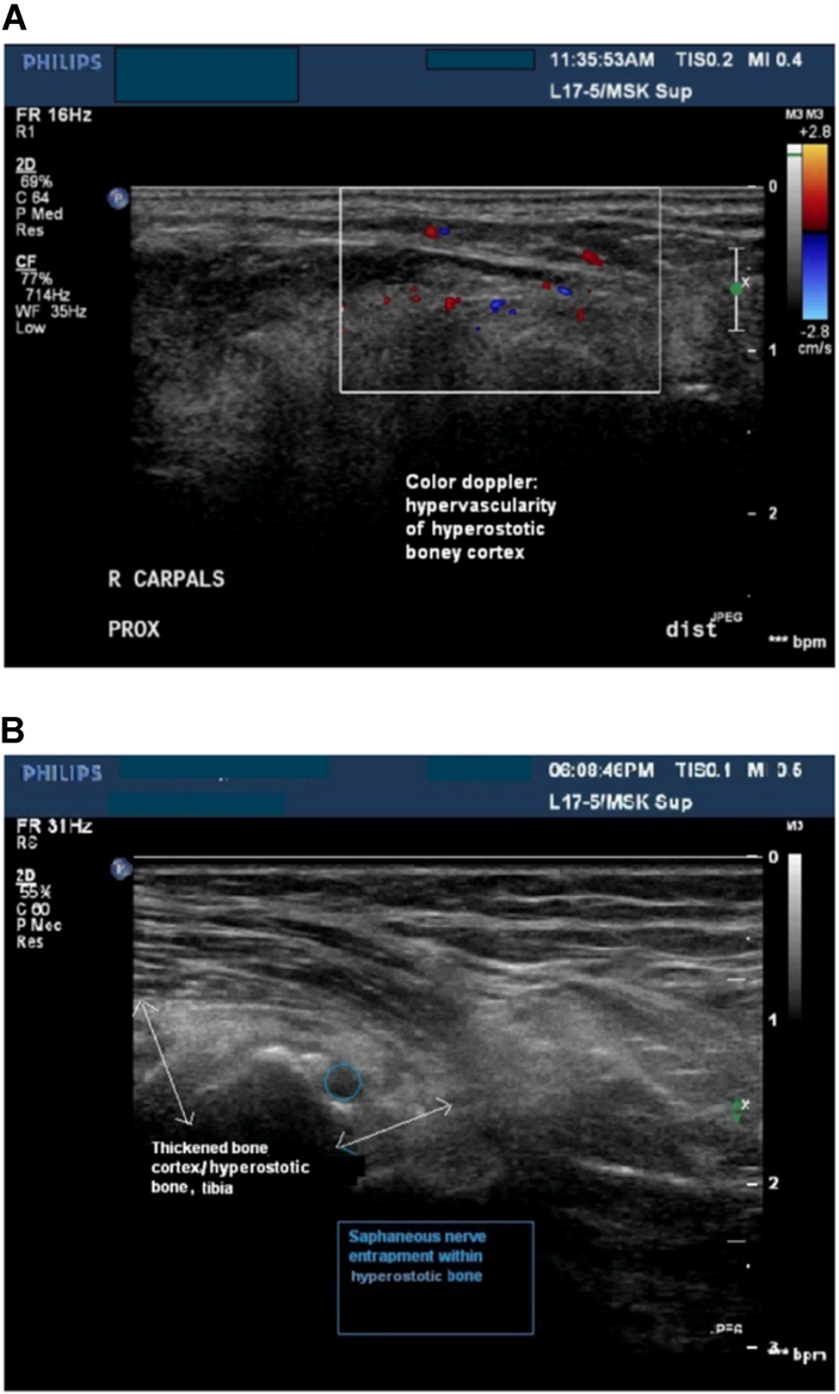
Neurologic consequences of melorheostosis. (*A*) Ultrasonogram imaging showing hypervascularity of the hyperostotic bone cortex (white box). (*B*) Ultrasonogram imaging (Melo‐10) showing hyperostotic bone (white arrows) with focal entrapment of the saphenous nerve (blue circle). The entrapped nerve was the likely cause of patient's pain, numbness, and tingling.
